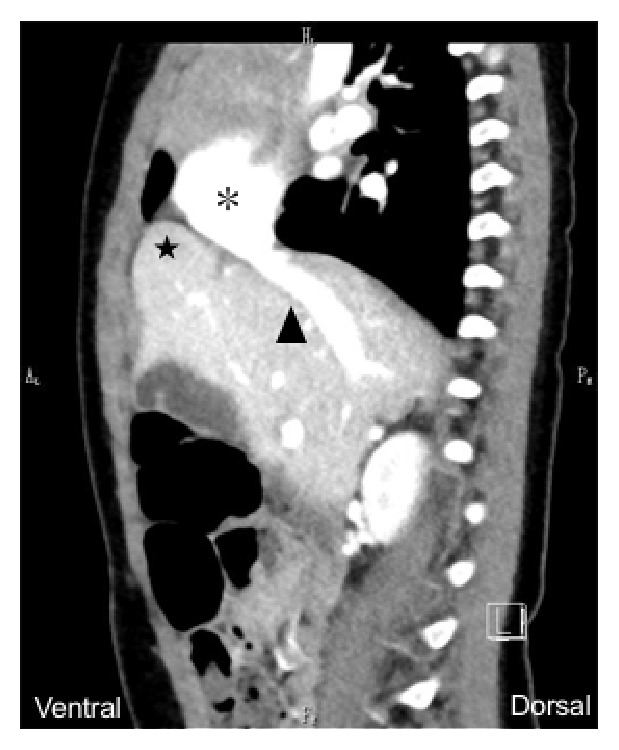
Abdominal computed tomography at 1 year of age. A Morgagni hernia (black star) can be seen, and the right atrium (asterisk) and inferior vena cava (arrowhead) are displaced on the ventral side.
